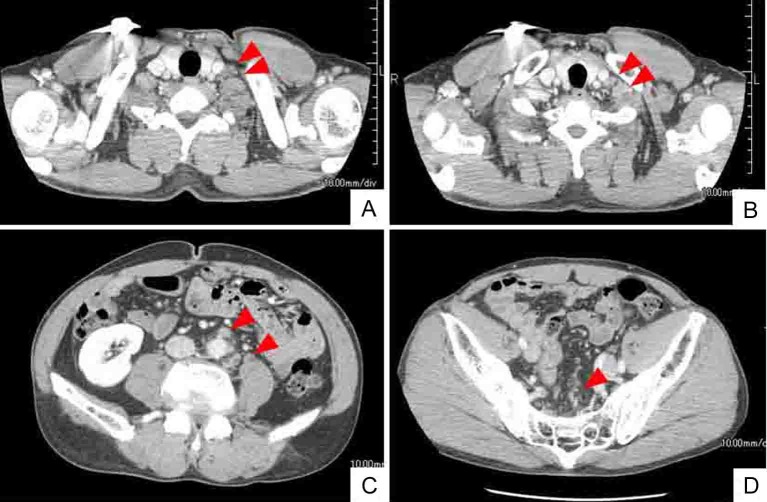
Whole body CT after 6 courses of chemotherapy (mFOLFOX6 and bevacizumab) shows smaller or disappeared metastatic LNs (arrow heads). Left cervical (A) and left supraclavicular (B) LNs. Abdominal periaortic, inferior mesenteric and surrounding (C), and regional colic (D) LNs.
